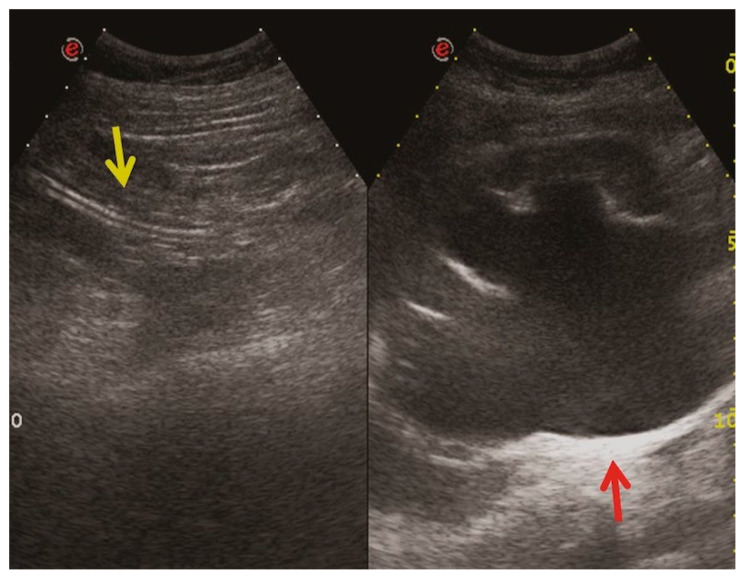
Ultrasound. Nephrostomy catheter seen outside the excretory tract within the retroperitoneal fat (yellow arrows). Marked hydronephrosis persists (red arrow).
